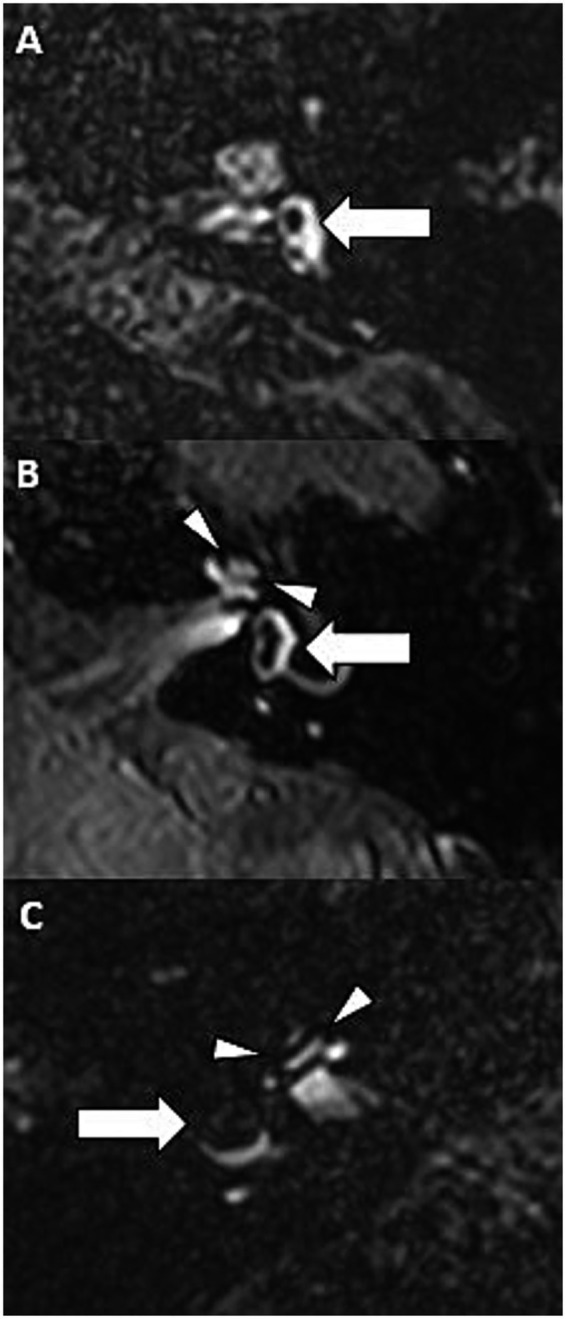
Examples of EH grading. Delayed post-gadolinium axial 3D T2 inversion recovery (A,C) and delayed post-gadolinium axial 3D FLAIR images. (A) There is enlargement of the saccule on the left (white arrow) without fusion with the utricle, consistent with a vestibular endolymphatic hydrops grade 1. (B) Asymmetrical perilymphatic enhancement, more pronounced on the left. Nodular hypo-intensities in the periphery of the apical and midturn of the cochlea on the left (arrow heads). No bandlike hypo-intensities in the basal turn of the cochlea making this a cochlear hydrops grade 1. The left saccule is enlarged and is just confluent with the utricule with a remaining peripheral rim enhancement, making this a vestibular hydrops grade 2. (C) There is fusion of the utricle and saccule on the right and the perilymphatic enhancement is no longer visible (arrow), compatible with a vestibular hydrops grade 3. The scala vestibuli is fully obliterated due to the distended cochlear duct (arrow heads), cochlear hydrops grade 2.
